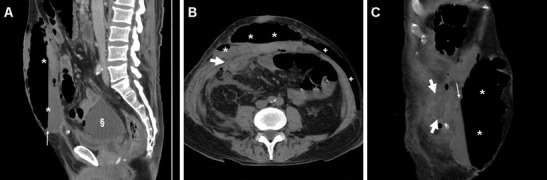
Surgically confirmed iatrogenic injury to the small bowel from laparoscopic VHR in a 76-year-old male. Sagittal (a), axial (b), and oblique coronal (c) images from contrast-enhanced early postoperative multidetector CT showed abundant fluid (§) and some air in the peritoneal cul-de-sac consistent with peritonitis, in communication (thick arrows) with a vast collection (*) with mixed content and air-fluid level (thin arrows) at the anterior abdominal wall
